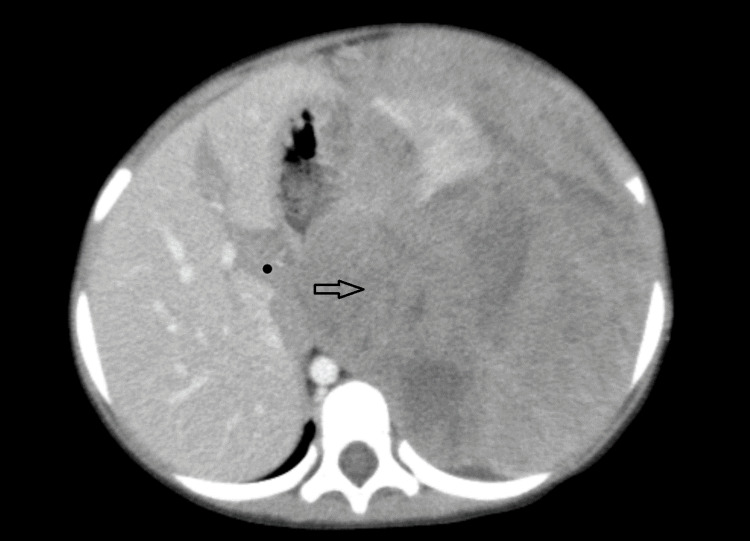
Axial CT image (19-02-2024) showing a left hemiabdomen mass. CT, computed tomography.
